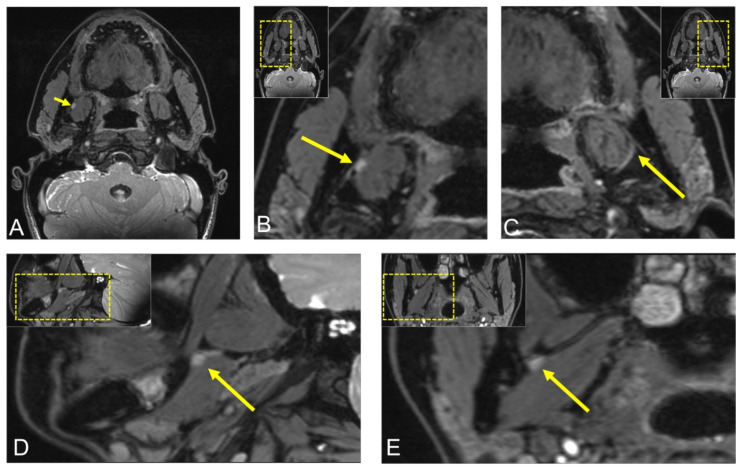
Postoperative visualization of the lingual nerve (LN) using 3D double-echo steady-state (3D-DESS) MRI in the same patient. (A,B) Axial, (D) sagittal, (E) coronal reconstruction of the region of interest displaying the LN asymmetry throughout its course through the infratemporal fossa, with an LN hyperintense nodular lesion of 5 × 3 mm between the right medial pterygoid muscle and the mandible. (C) Axial image reconstruction of the healthy LN on the contralateral side for comparison. For orientation, the dotted rectangles in the corner show the enlarged area.
